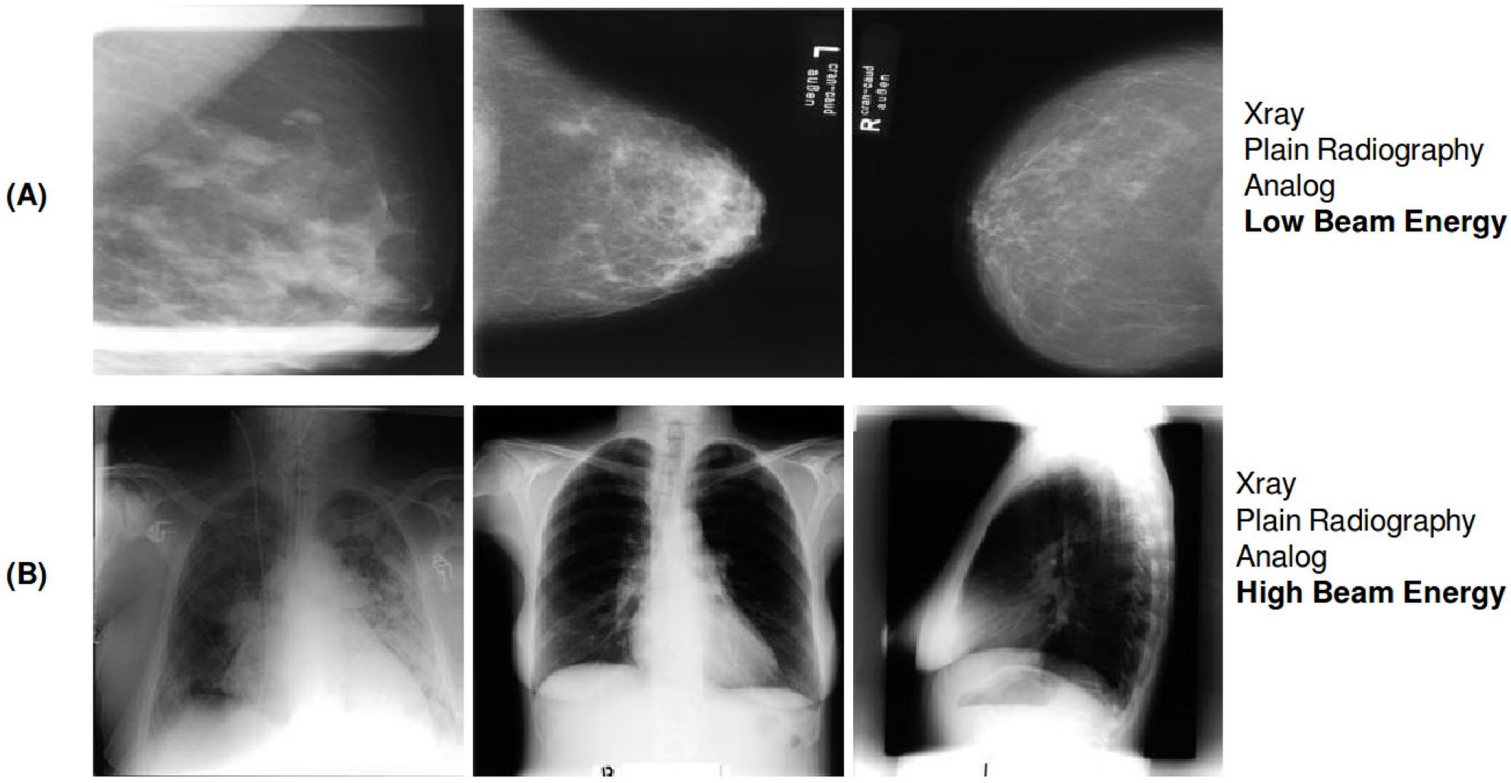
Examples of radiographs annotated with two classes from the T-scheme. (A) shows three images belonging to class ‘1124’ representing ‘Xray; Plain Radiology; Analog; Low Beam Energy’ and (B) displays three images belonging to class ‘1123’ representing ‘Xray; Plain Radiology; Analog; High Beam Energy’. All radiographs were randomly chosen from the ImageCLEF 2009 Medical Annotation Task Training Set. Republished from [21] under a CC BY license, with permission from [RWTH Aachen], original copyright [2009].
